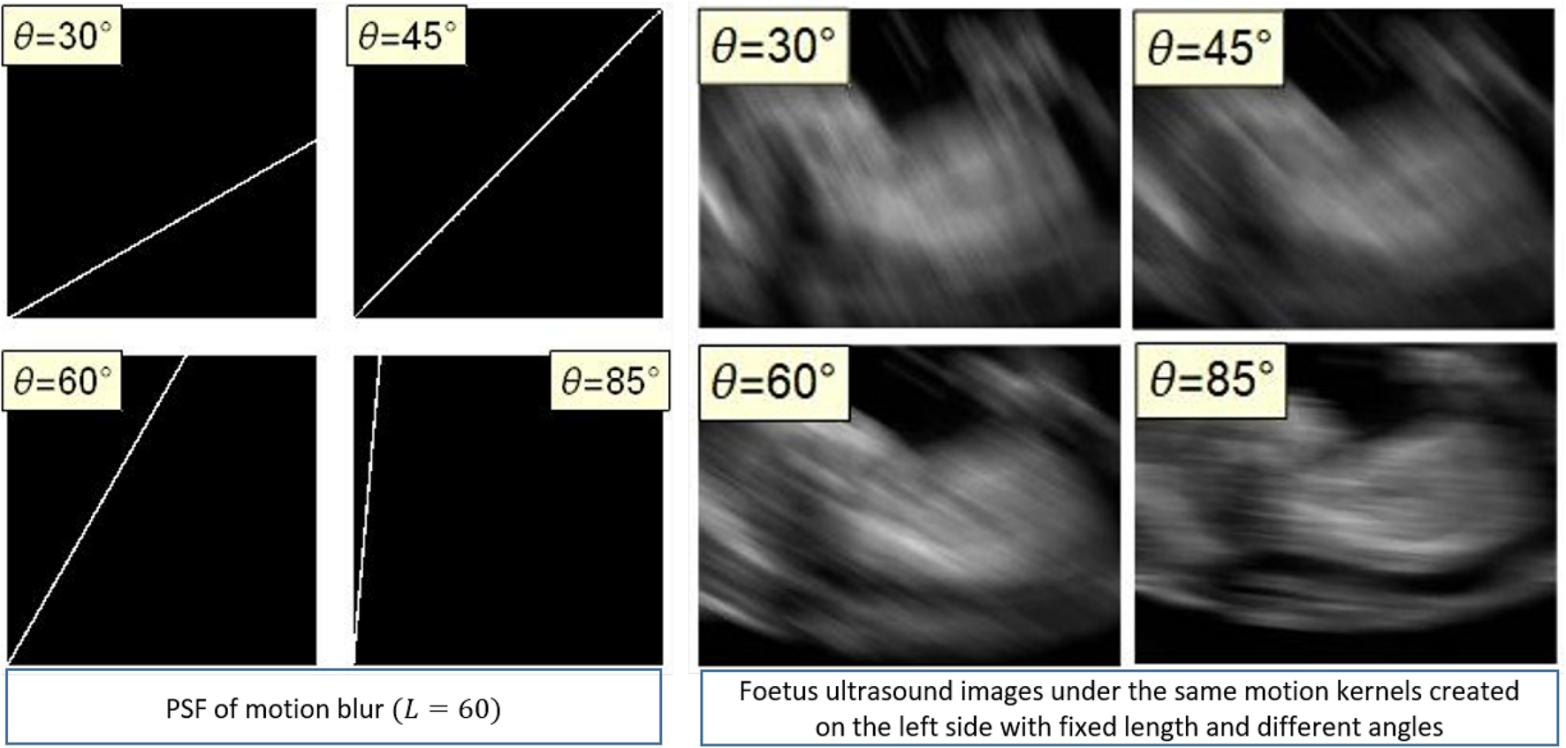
Understanding of motion blur. Left: PSF of motion blur in spatial domain for $$L=60$$ and different angles $$(\theta =30^{\circ },45^{\circ },60^{\circ },85^{\circ })$$ and right: Fetus ultrasound images with fixed length and different angles of motion.
